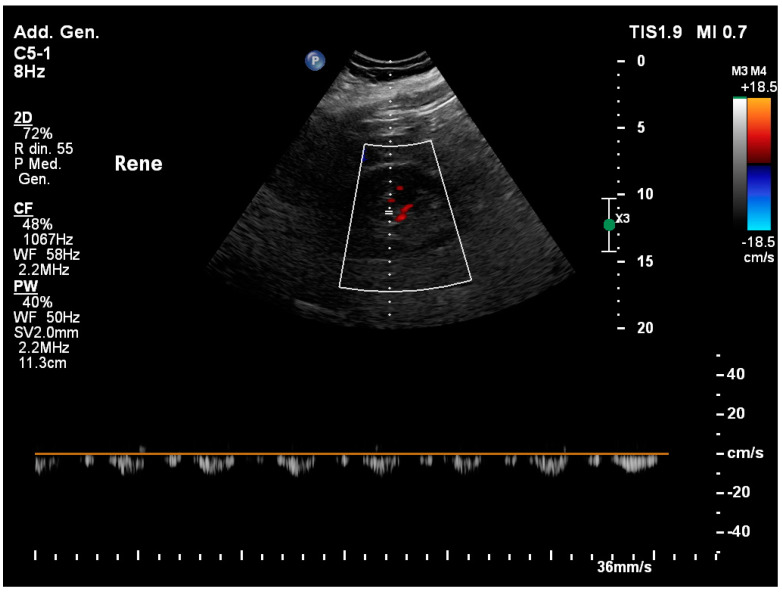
The diastolic phase is crucial in the ultrasound assessment of renal venous flow. When congestion occurs, the two components S and D turn discontinuous and pulsatile. At the most extreme levels of congestion, the two components are undistinguishable, like one single diastolic peak.
